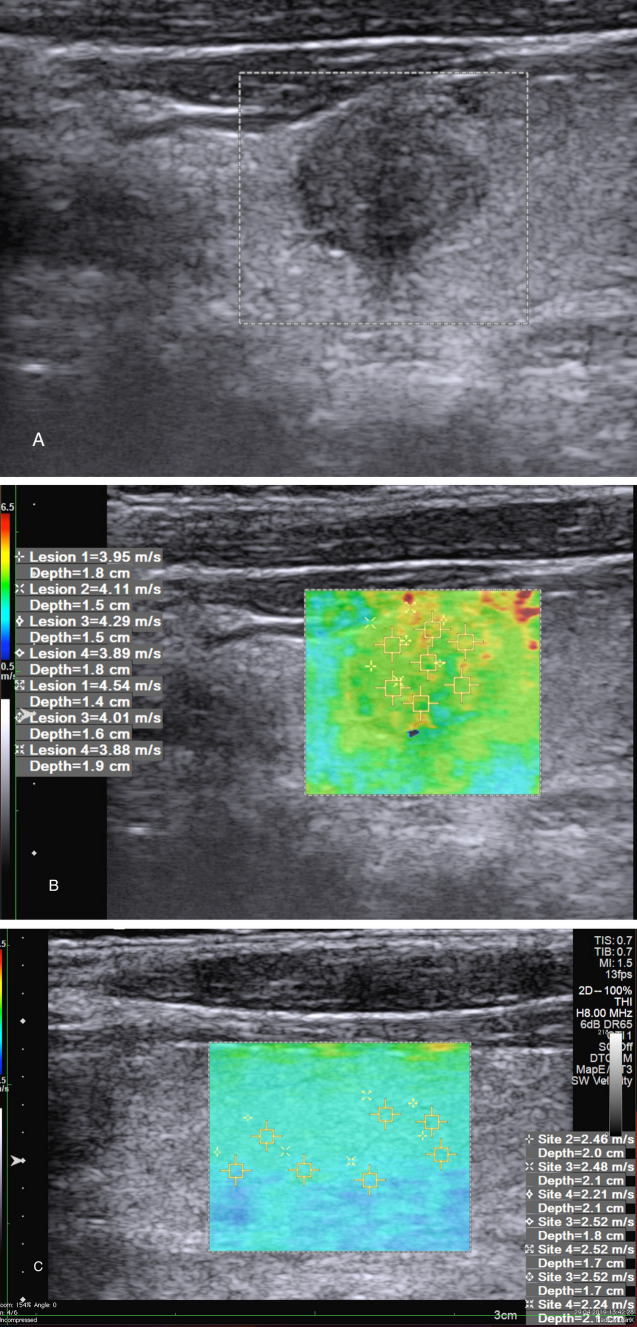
A 58 year old female patient with surgically proven papillary carcinoma. A, B-mode ultrasound shows a hypoechoic solid nodule with slightly irregular margin in the left thyroid gland, upper pole. B, Shear wave image with thyroid nodule. SWV measurements of nodule was as 4.09 m/s and C, for thyroid tissue was 2.42 m/s
